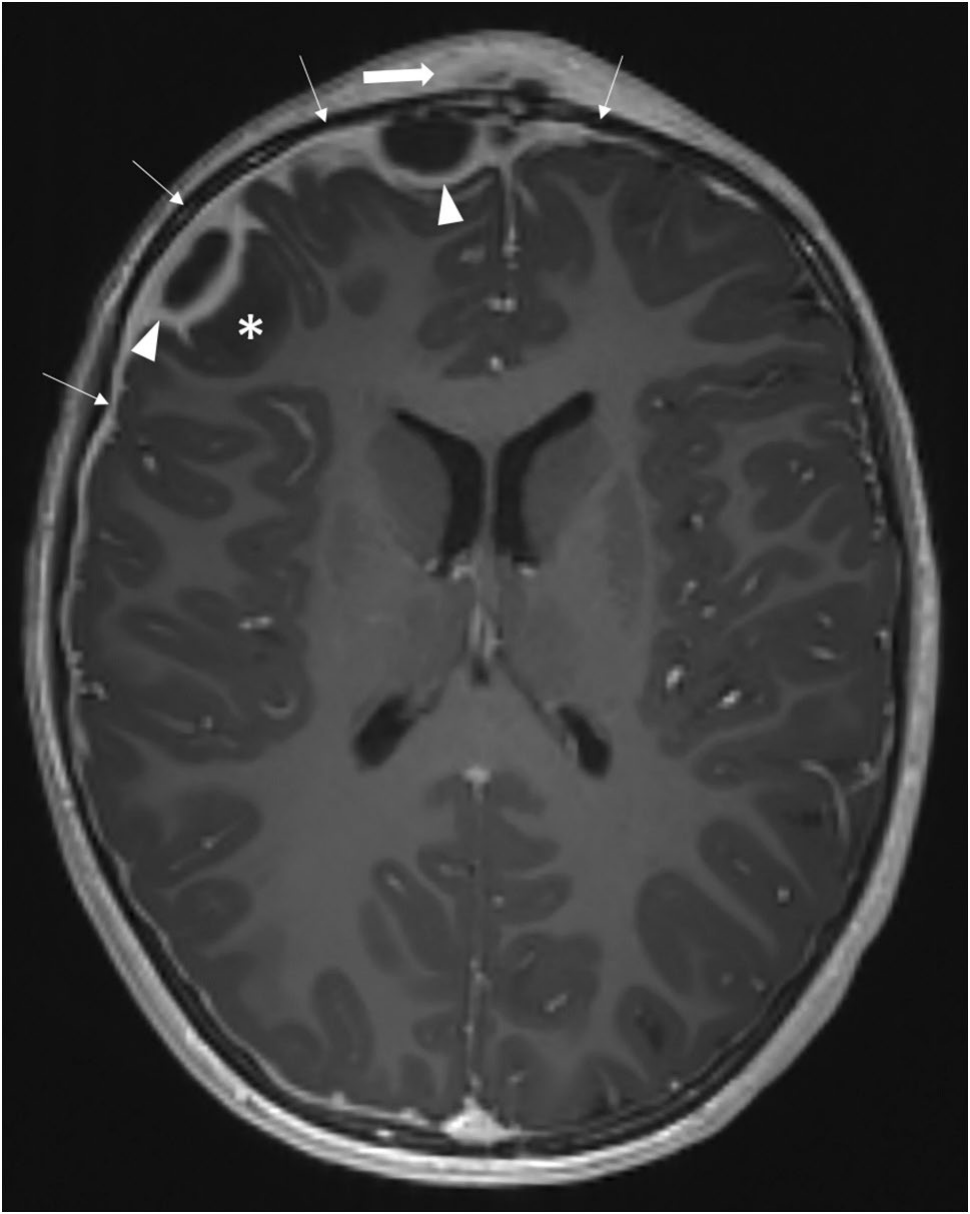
Axial T1-weighted magnetization prepared-rapid gradient echo sequence magnetic resonance image after intravenous contrast agent administration in an 8-year-old boy with osteomyelitis of the frontal bone as a complication of acute sinusitis of the frontal sinus and ethmoidal cells. Subperiosteal abscess on the forehead (Pott’s puffy tumor, *thick arrow*) and frontal epidural and subdural abscesses (*arrowheads*) are visible as well as accompanying cerebritis of the right-sided gyrus frontalis inferior (*asterisk*) and accompanying meningeal reaction (*thin arrows*)
